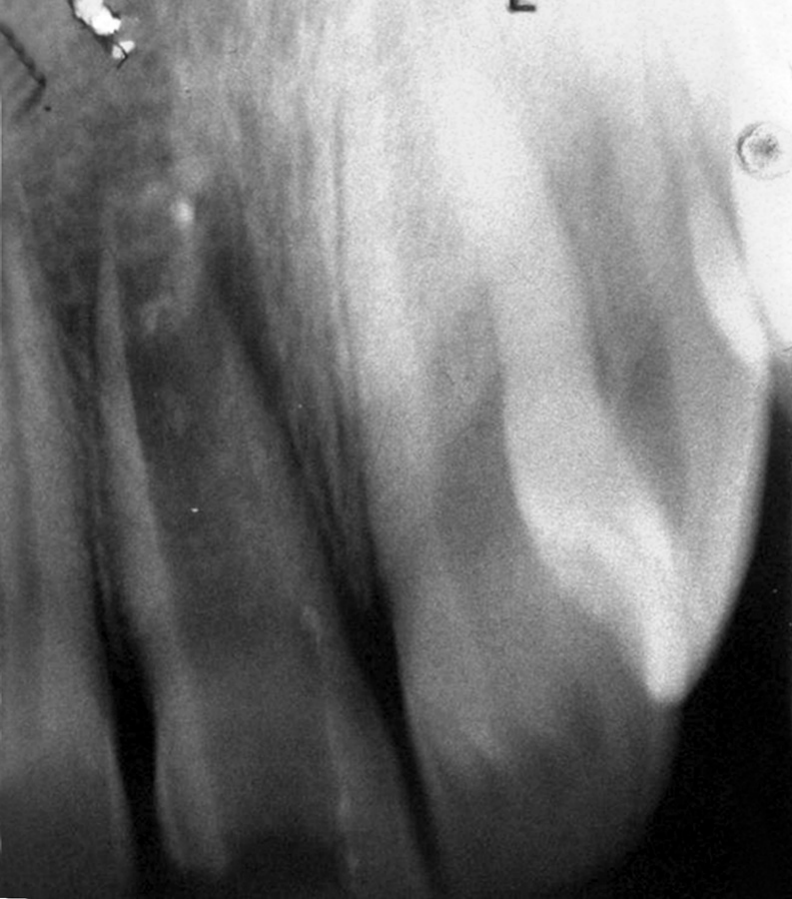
Preoperative radiograph of right central incisor
